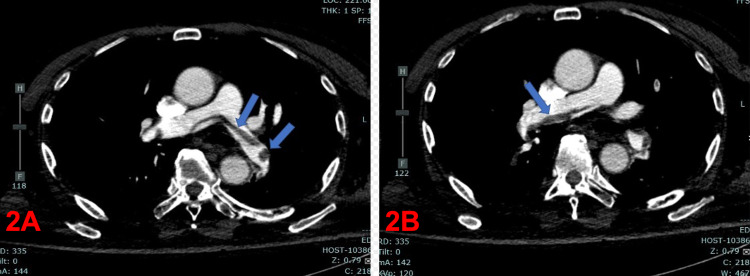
Computed tomography angiography pulmonary embolus series showing saddle embolus. A: Blue arrows showing thrombus occluding the left pulmonary artery. B: Blue arrow showing thrombus occluding the right pulmonary artery.
